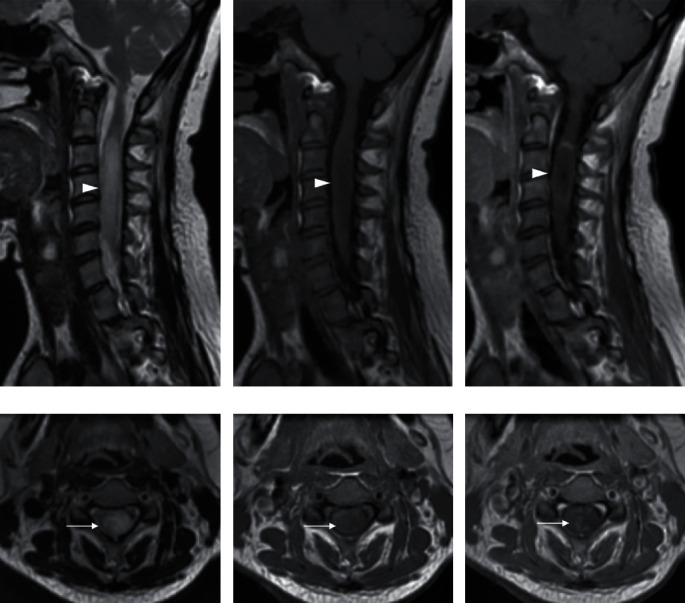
Cervical spinal cord MRI of an NMO patient in our center, a 32-year-old female, with left-sided hemiparesis for two weeks, showing (a) T2WI-hyperintense longitudinally extensive myelitis, spanning across more than 3 vertebral segments; (b) hypointense on unenhanced T1WI; and (c) with peripheral enhancement on contrast-enhanced T1WI (arrowheads). Corresponding axial (d) T2WI, (e) unenhanced T1WI, and (f) contrast-enhanced T1WI, showing the involvement of more than 50% of the cord surface area (arrows).
